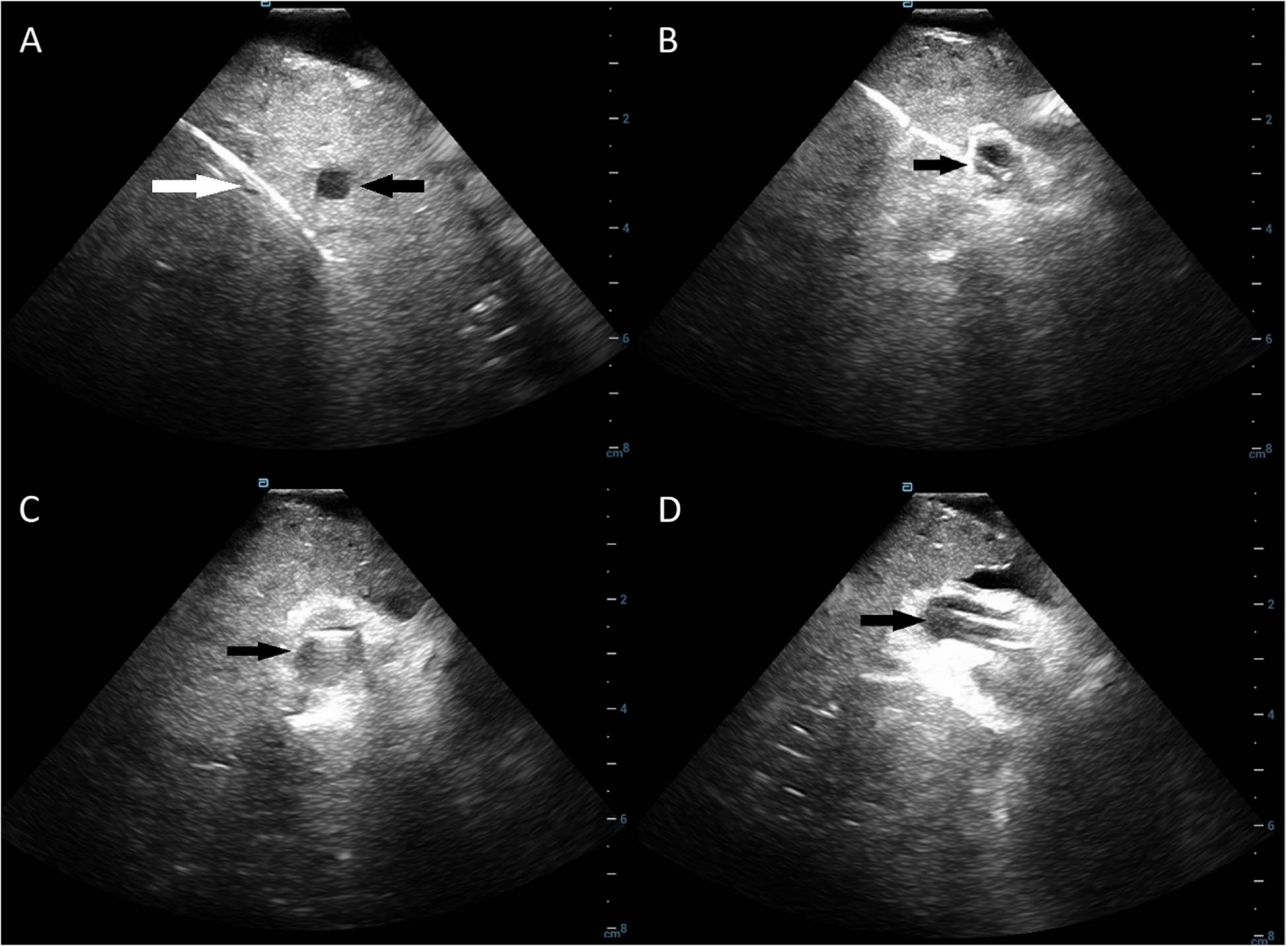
Portal Vein Access Under Intracardiac Echocardiography (ICE) Guidance. A US visualization of right hepatic vein catheterization (white arrow) with adjacent right portal vein (black arrow). B Advancement of the ICE catheter into the right portal vein (black arrow). C TIPS needle tip (black arrow) in the portal vein. D Advancement of the TIPS introducer sheath into the portal vein (black arrow)
